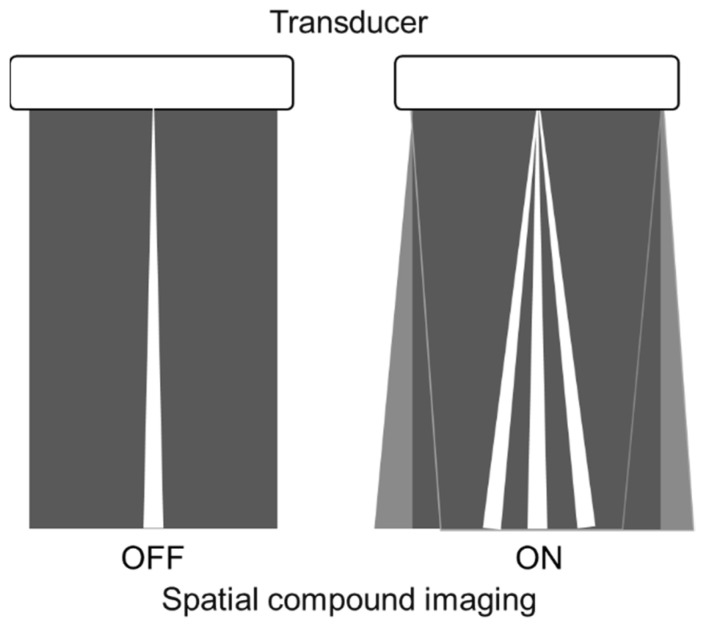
Spatial compound imaging is a method wherein sonographic information is obtained from several different insonation angles and combined to produce a single image.
